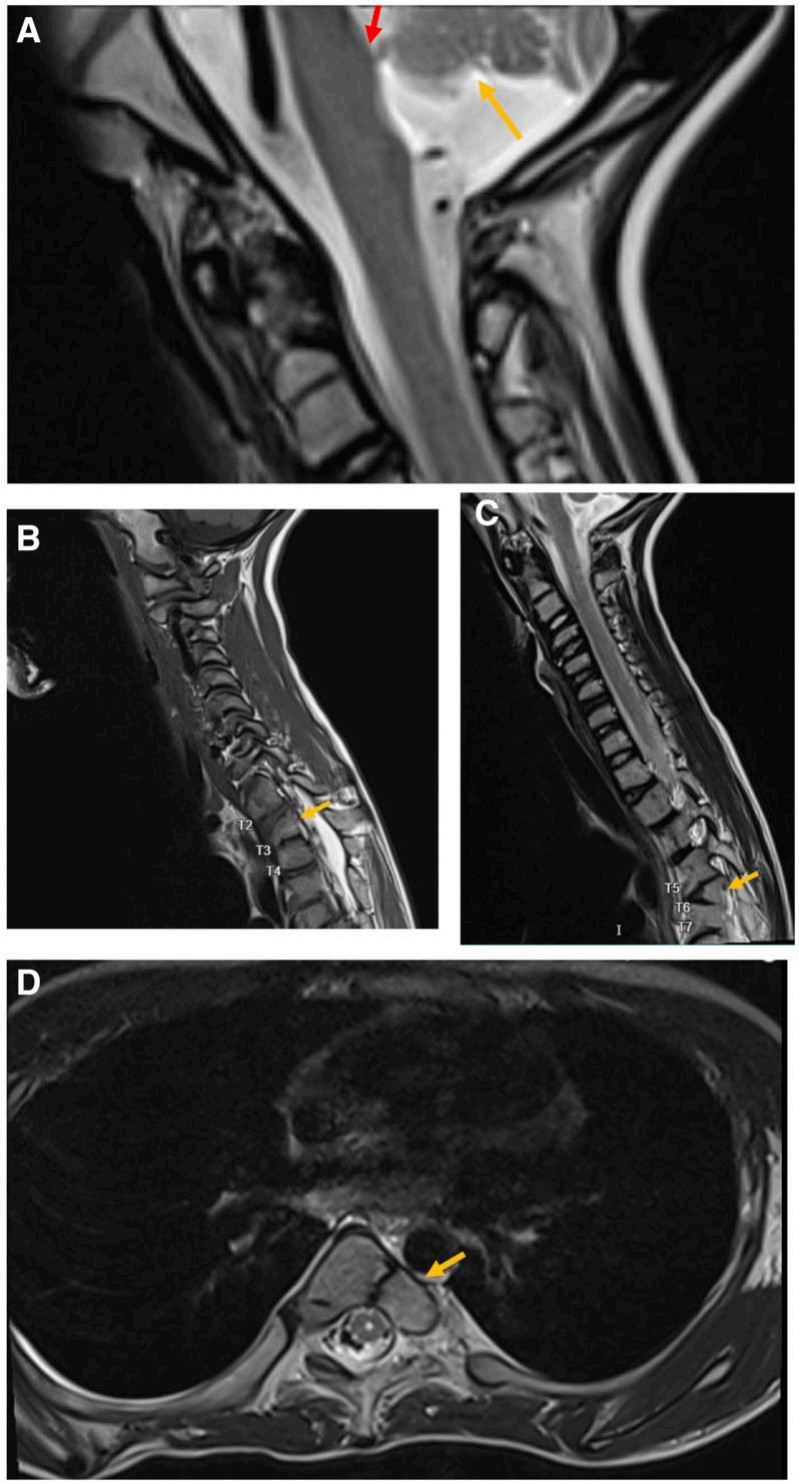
A-D, MRI Spine Cervical-Thoracic-Lumbar without contrast sagittal view T1 weighted. A, The inferior arrow displays partially visualized hypoplasia of the cerebellar peduncles and vermis. The superior arrow demonstrates the pontine tegmentum bulging into the fourth ventricle representing a cap. B, The arrow shows right hemivertebra of T3. C, The arrow shows the butterfly hemivertebra of T6. D, MRI Spine Cervical-Thoracic-Lumbar without contrast axial view T2 weighted. The arrow shows the axial plane through the hemivertebra.
